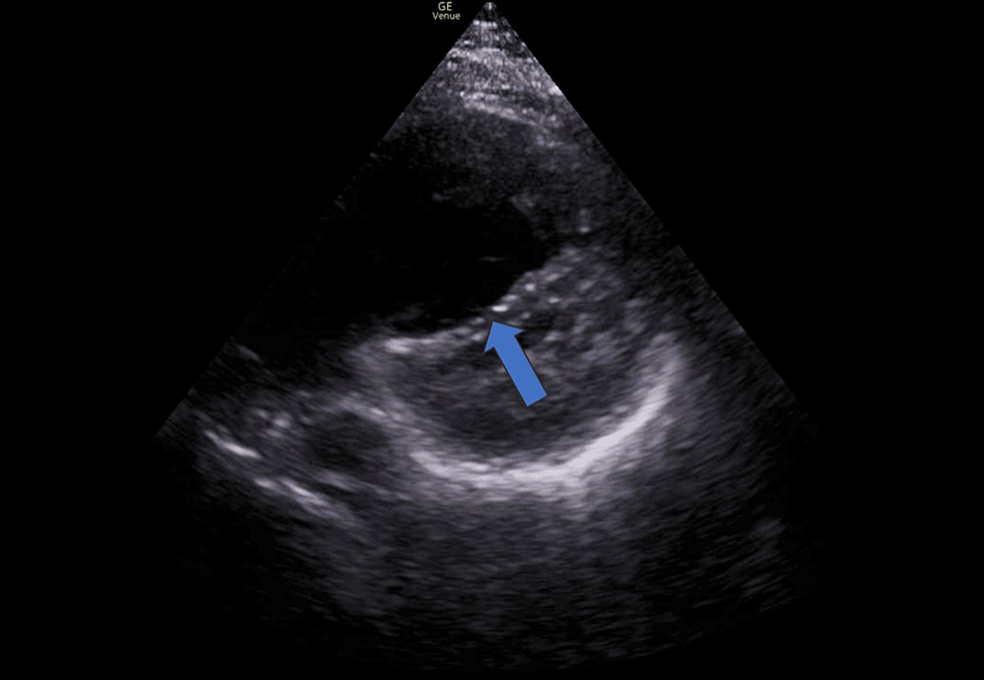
Point-of-care cardiac ultrasound showing right ventricular dilation and septal bowing
